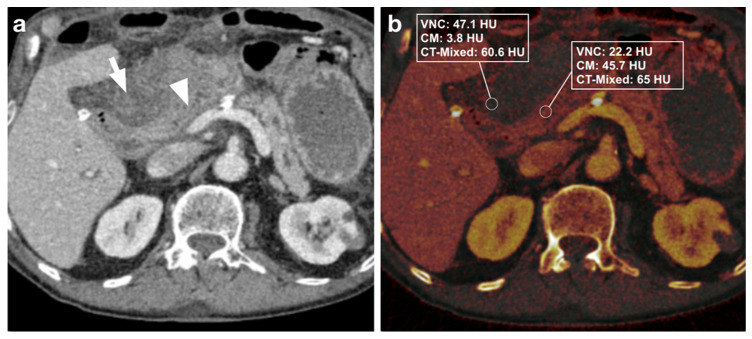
(a) 81-year-old male patient with intra-abdominal hematoma (arrow) adjacent to a small bowel loop (arrowhead) after Whipple surgery. (b) Measurement of iodine maps shows a difference in VNC and iodine values (dual-energy CT) between hematoma and intestinal loops, but no difference in DE-LB (single-energy CT). Due to the extensive hematoma, a re-laparotomy was subsequently performed.
